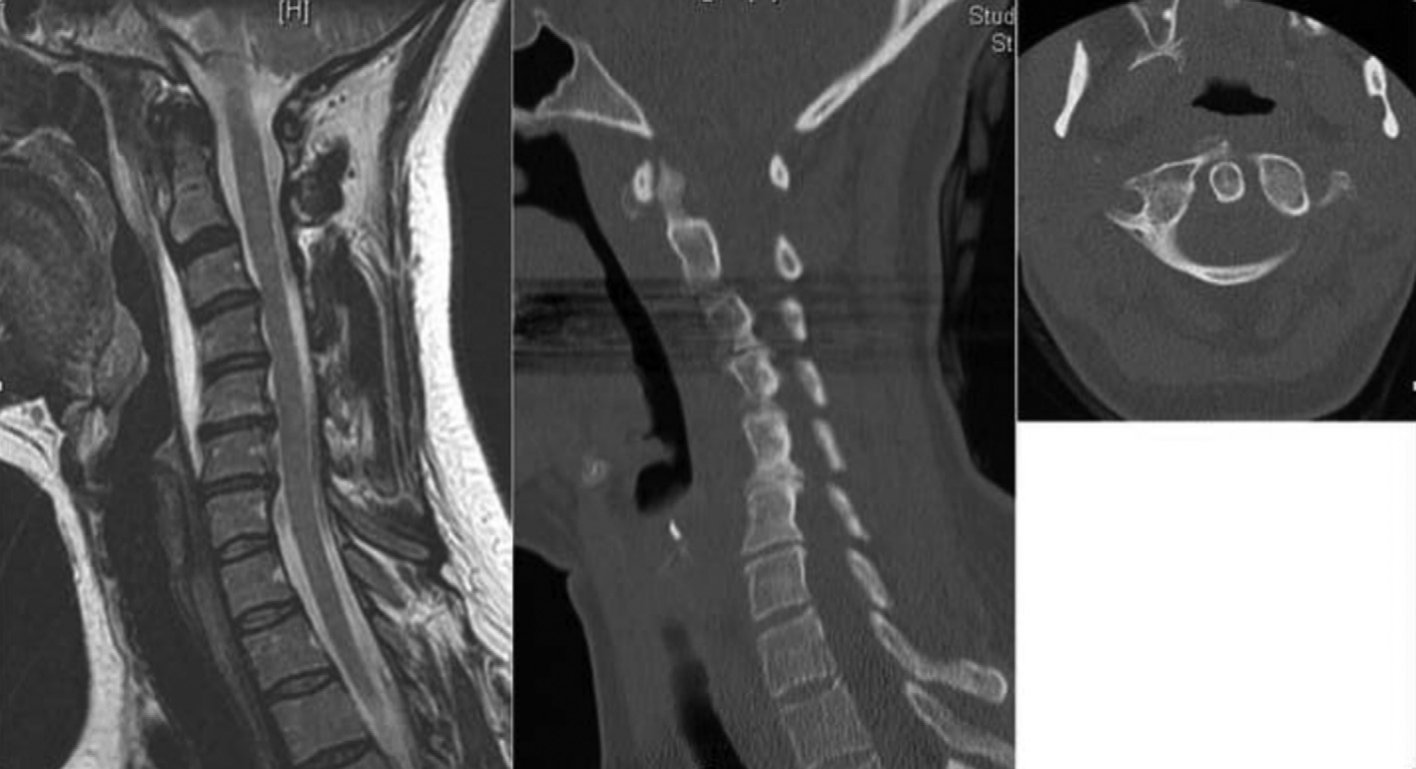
MRI and CT scans of another case showing loss of cervical lordosis, even kyphotic cervical spine, and prevertebral fluid collection extending from skull base caudally to C5, maximally anterior to C4. The CT scan shows the faint small calcification in front of C1, mainly on the right side.
